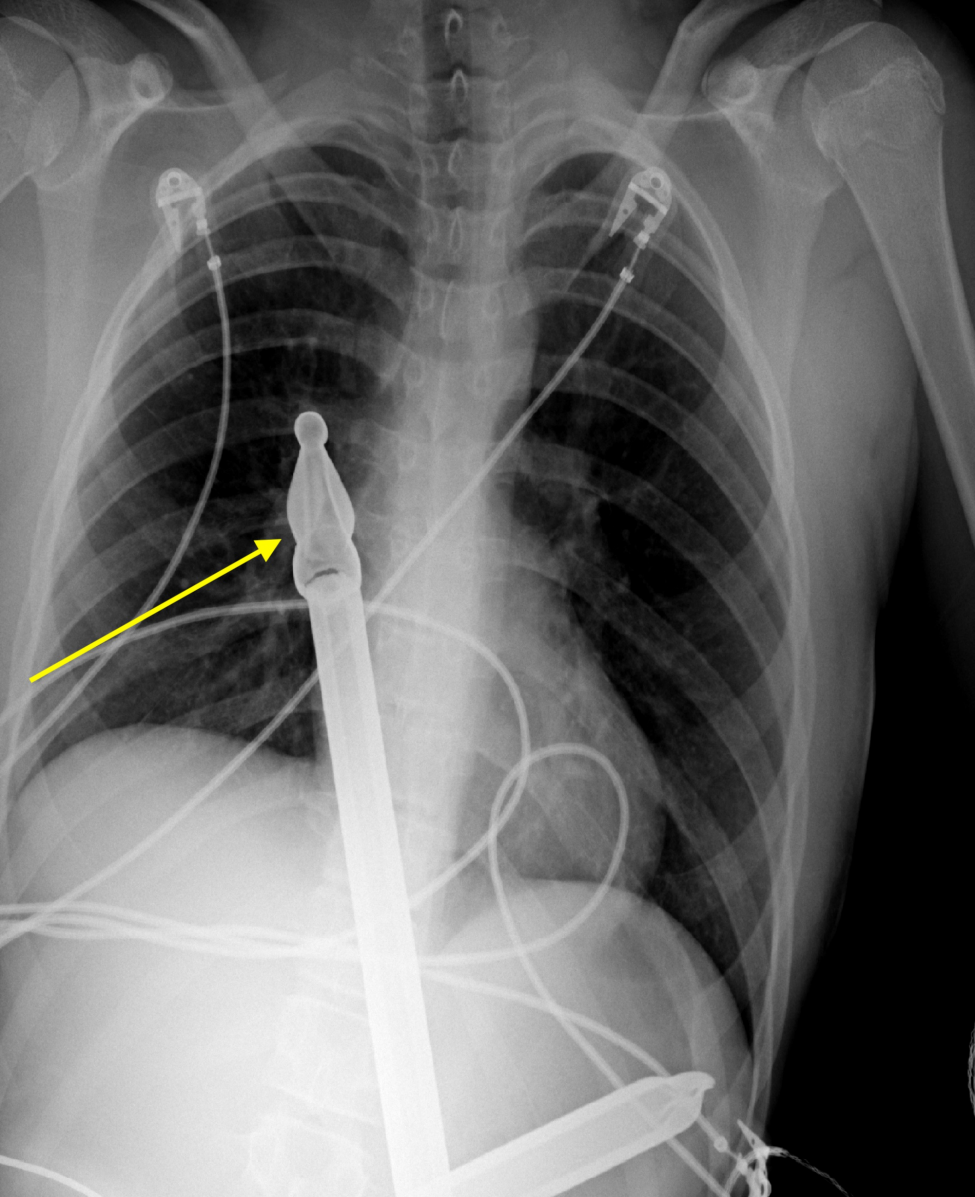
Frontal chest radiograph demonstrates a large foreign body (yellow arrow) consistent with a metal fence post projecting into the mid-right hemothorax
